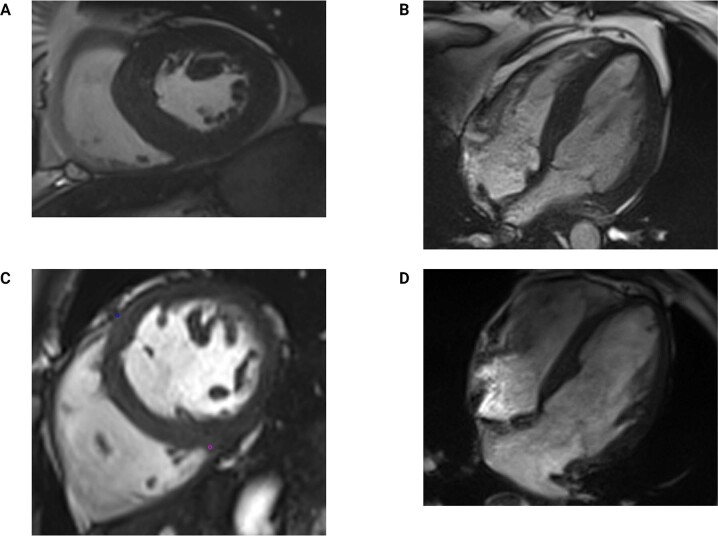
Cardiac magnetic resonance images demonstrating left ventricular hypertrophy and normal dimensions in dialysis patients. Short axis stack image (A) and horizontal long axis image (B) of a patient on haemodialysis with concentric left ventricular hypertrophy on cardiac magnetic resonance imaging (maximum wall thickness, 15 mm; left ventricular mass 93 g/m^2^). Short axis stack image (C) and horizontal long axis image (D) of a patient on peritoneal dialysis with normal left ventricular dimensions on cardiac magnetic resonance imaging (maximum wall thickness, 9 mm; left ventricular mass 63 g/m^2^).
